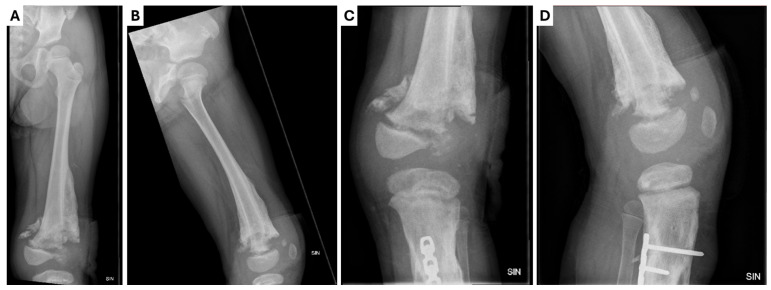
X-ray examination of the (A,B) left hip joint and (C,D) femur and knee joint in anterior–posterior and lateral projections showing a small, heterogeneous lateral condyle of the femur, a lytic zone in the metaphysis, massive calcification in the mid-diaphysis, a normal hip joint gap, smooth surfaces, and no pathologies in the hip joint bones.
